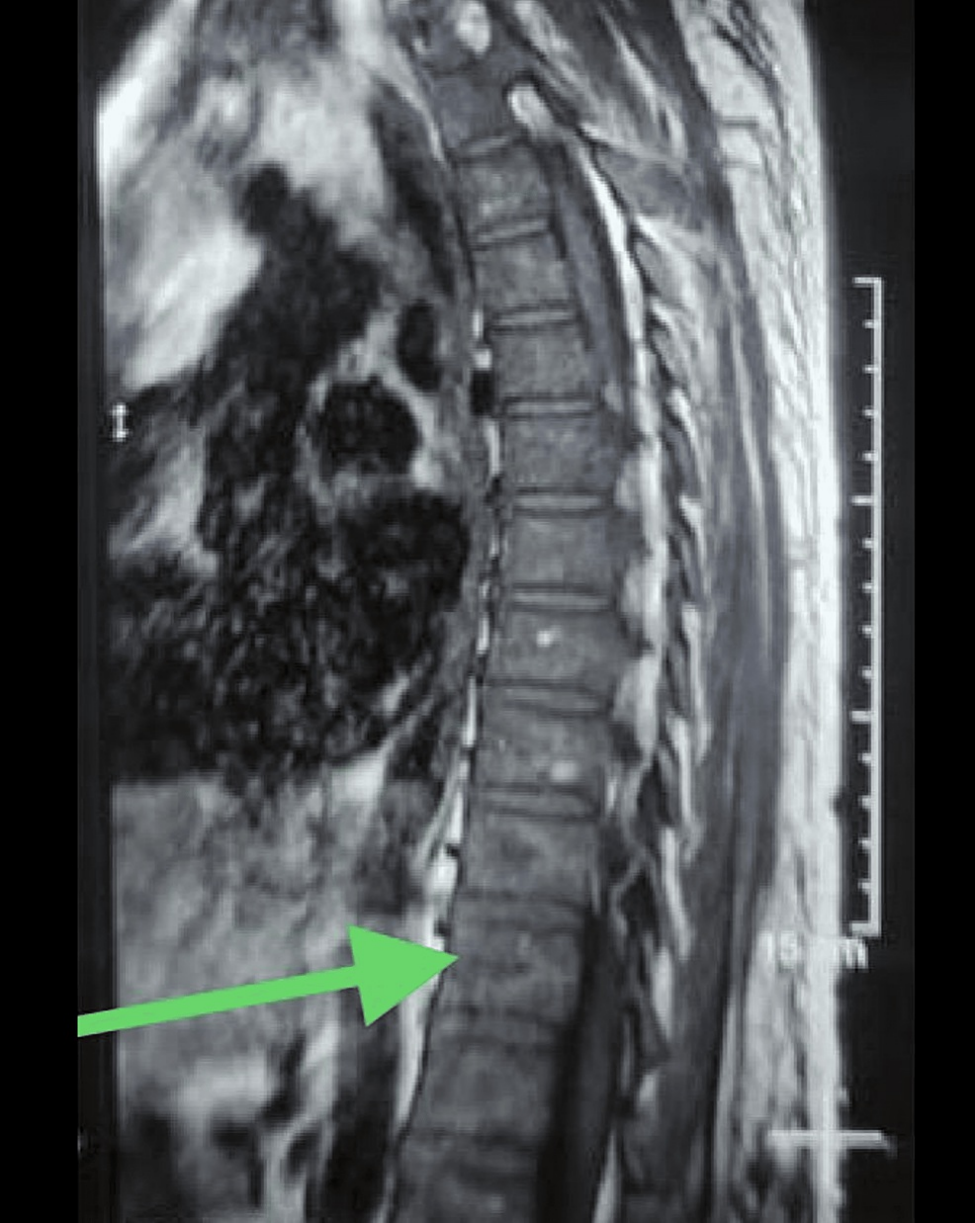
MRI of the spine showing the T2 and STIR sequences in the vertebral bodies and paravertebral soft tissue. MRI: magnetic resonance imaging, T2: transverse relaxation time, STIR: short tau inversion recovery
